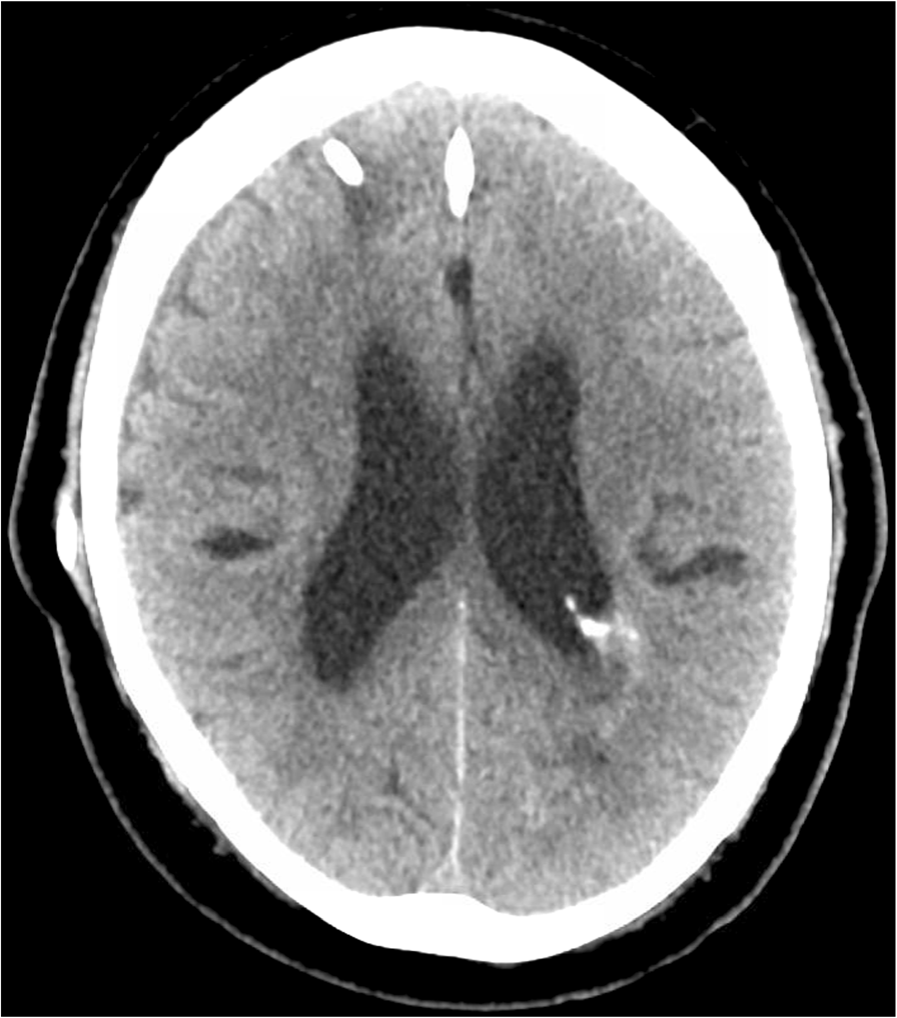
CT head post op day 25 after revision, showing resolution of hygroma and CM-related hemorrhage
